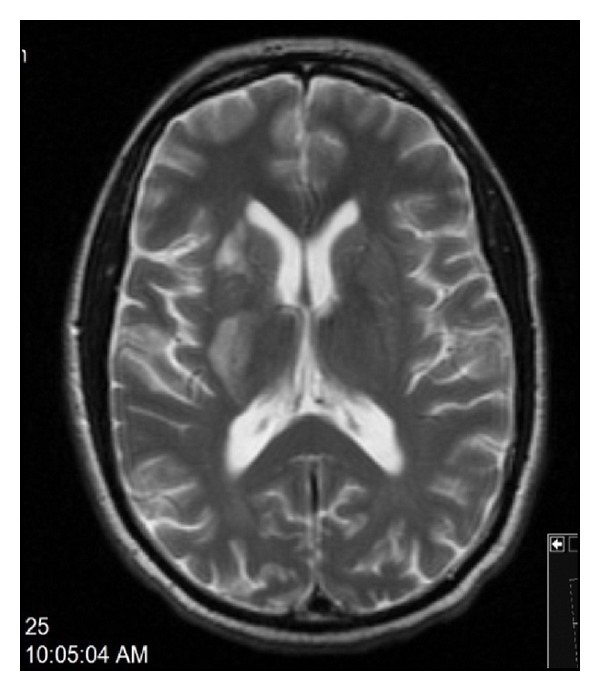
Brain MRI with gadolinium shows the infarct clearly.
